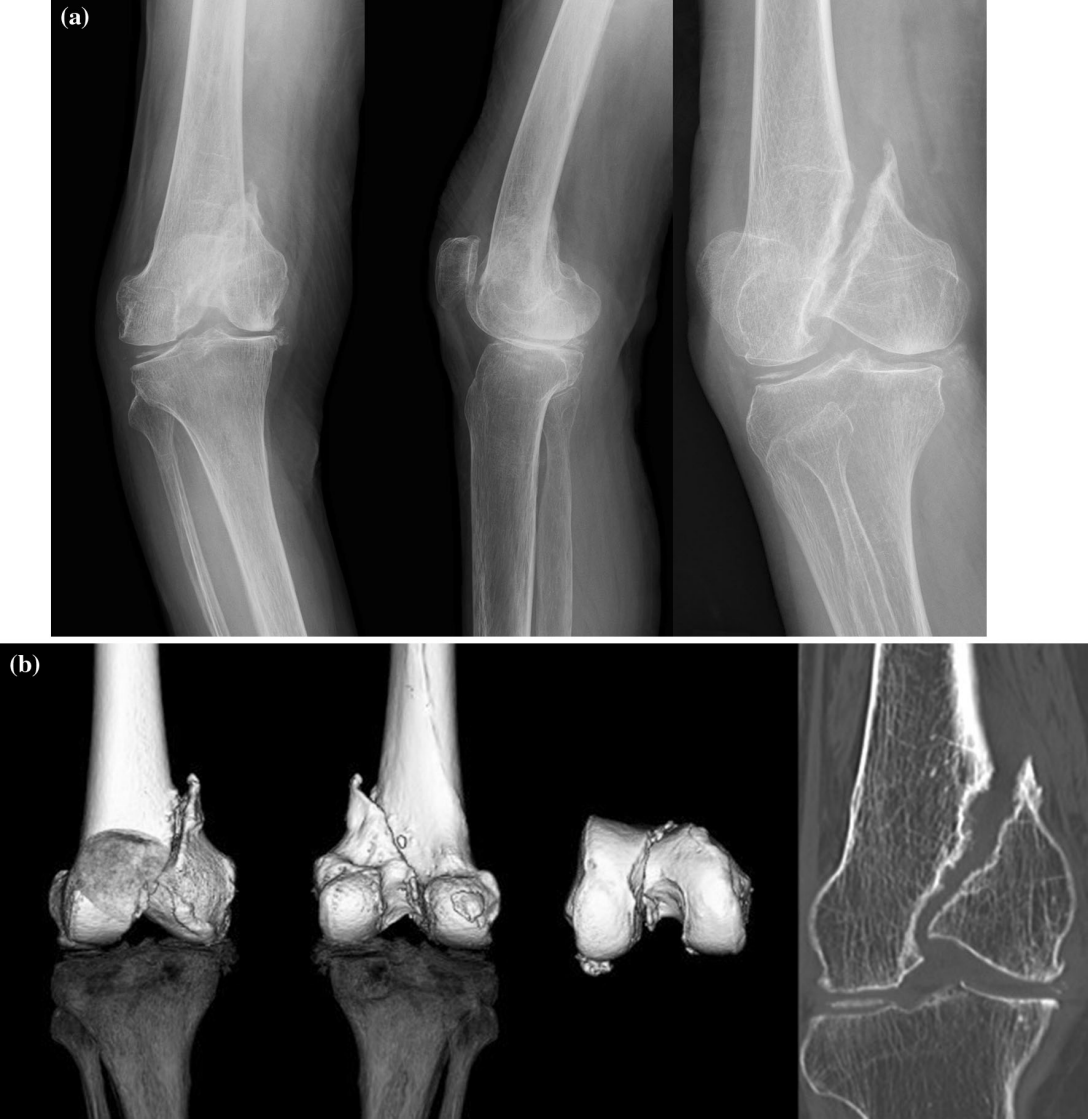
a Radiographs demonstrating medial condyle fracture nonunion of the femur. b computed tomography demonstrating the details of the fracture nonunion
